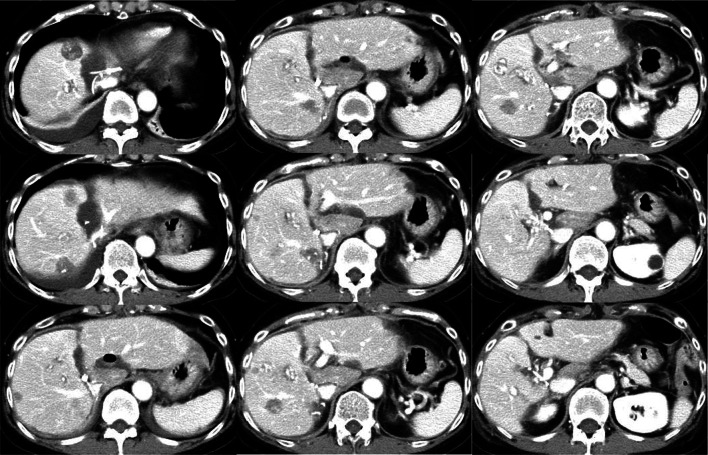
Computed tomographic findings preceding stage 2 of ALPPS. No ischemia or congestion is evident, although blood supply appears slightly decreased within the left caudate lobe
